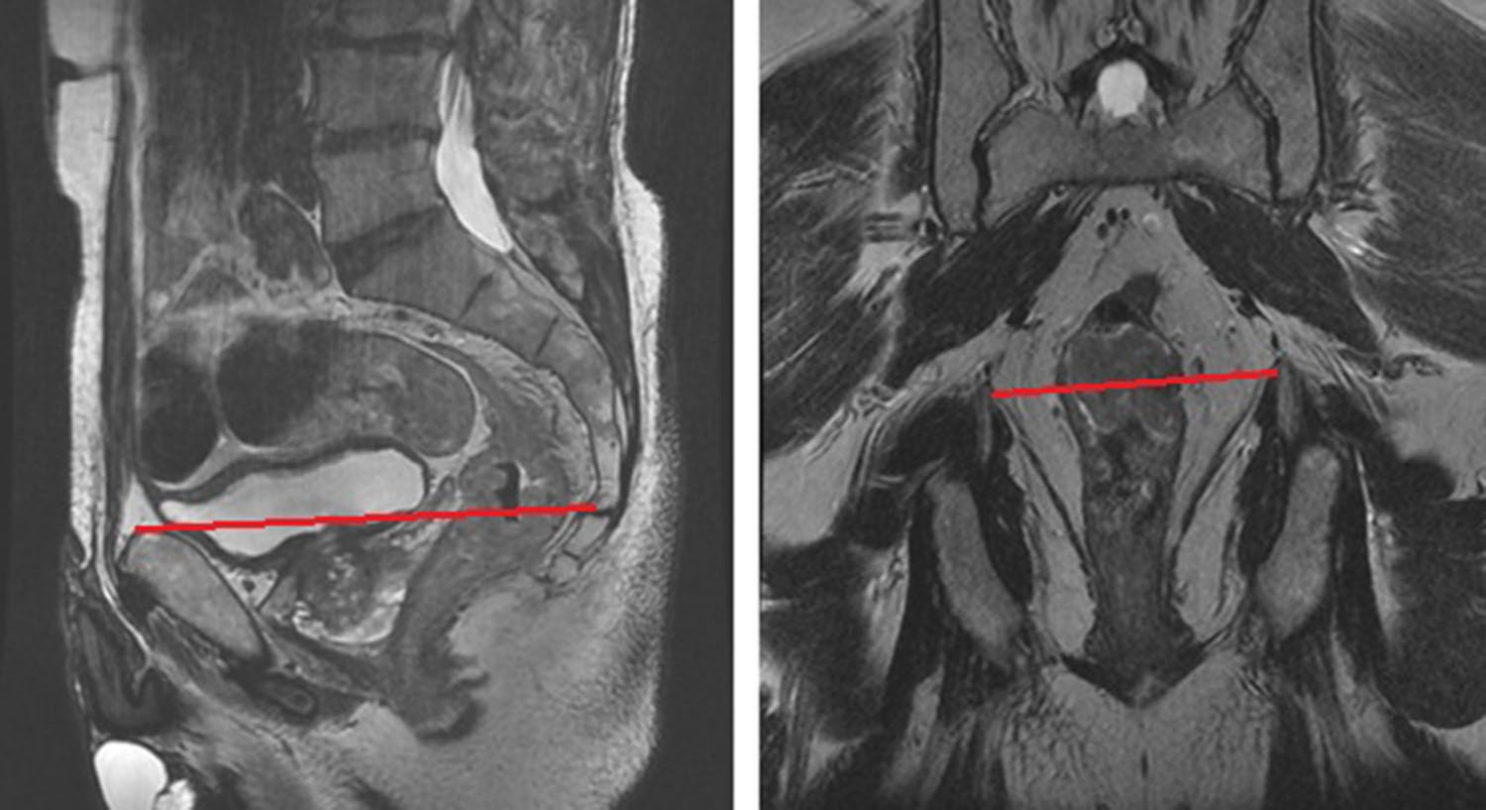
MRI definition of a low rectal tumor (sagittal (left) and coronal (right) T2 MRI-scans showing the line between the pubic bone and the origin of the levator muscles: a tumor below this line was defined as a low rectal cancer)
